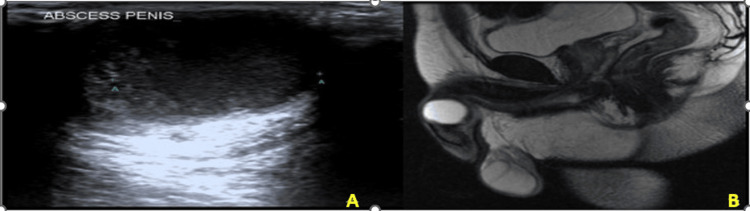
Imaging Representation of Penile Abscess (A) Ultrasound image showing a well-defined fluid collection of size 3.0 x 2.0 x 2.7 cm predominantly on the left side of the cavernosa. (B) MRI showing a well-defined lesion with stretching of the tunica albuginea and displacing the spongiosum.
